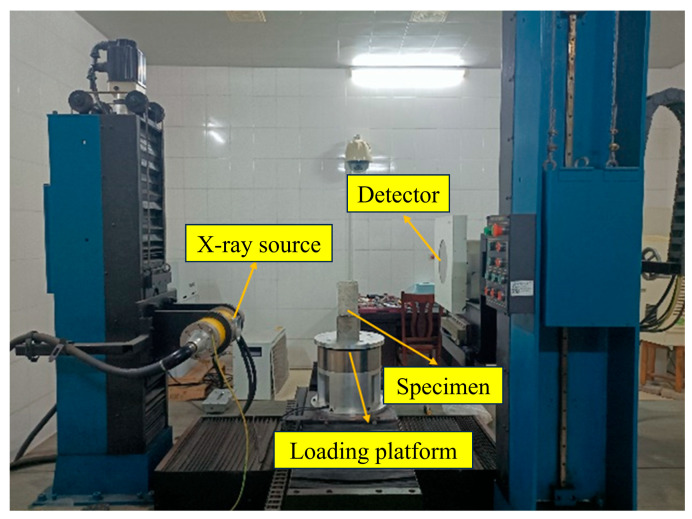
Industrial CT scanning equipment.
